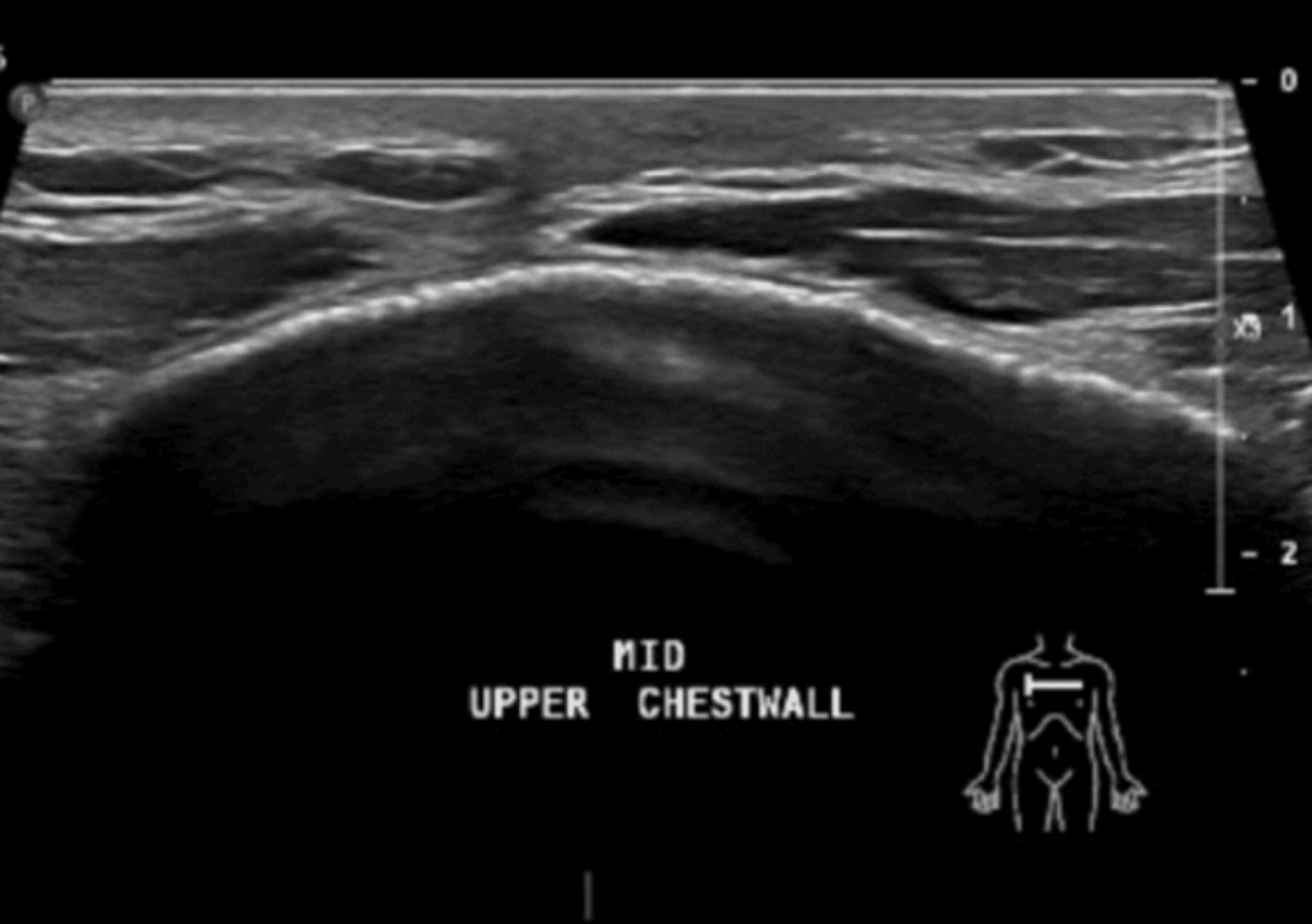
Normal chest wall ultrasound
